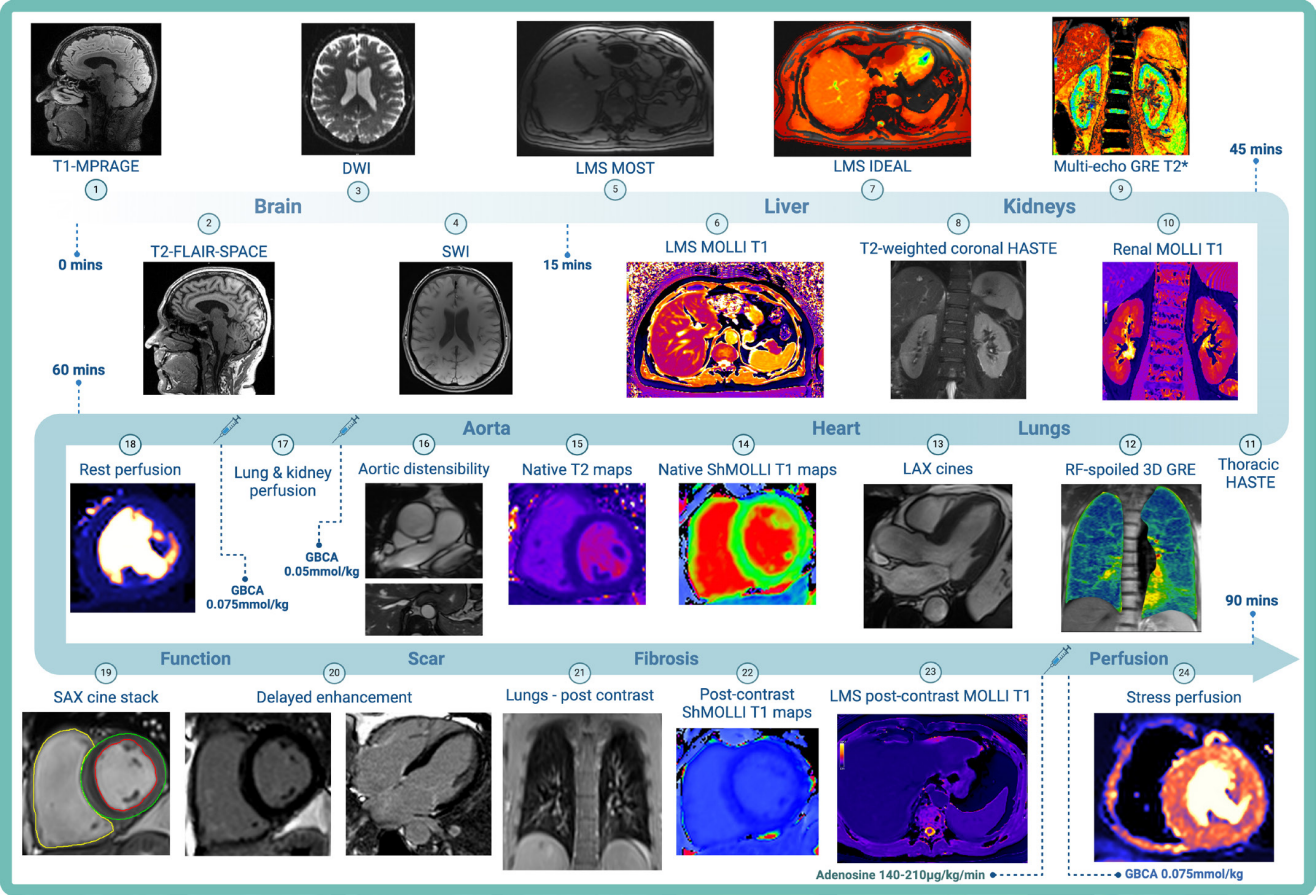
COSMIC multiorgan MRI protocol. DWI, diffusion-weighted imaging; GBCA, gadolinium-based contrast agent; GRE, gradient echo; HASTE, half-Fourier acquisition single-shot turbo spin echo; IDEAL, iterative decomposition of water fat with echo asymmetry and least-squares estimation; LAX, long axis; LMS, liver multi-scan; MOLLI, modified Look-Locker inversion recovery; MOST, magnitude only thin-slice T2*; MPRAGE, magnetisation-prepared rapid acquisition with gradient echo; RF, radiofrequency; SAX, short axis; ShMOLLI, shortened modified Look-Locker inversion recovery; SWI, susceptibility-weighted imaging; T2-FLAIR, T2-weighted fluid-attenuated inversion recovery; 3D, three-dimensional.
